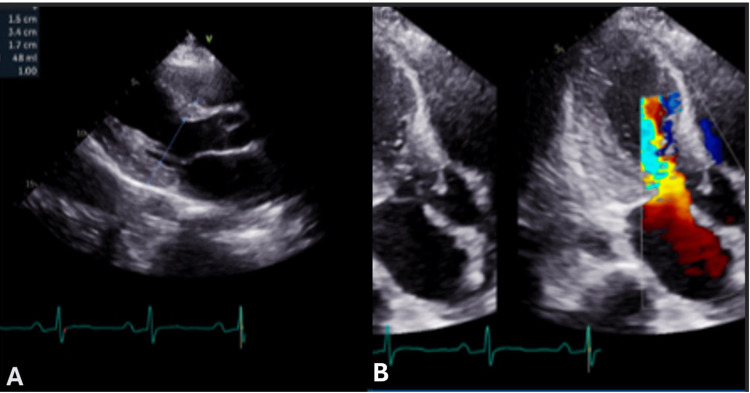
Parasternal long axis showing a septum and LV measurement of 1.5 cm consisting of LVH (A). Apical long axis showing an EF of 60%, diastolic dysfunction with increased mean LA pressure, and systolic anterior motion with LVOTO with a peak gradient of 111 mmHg (B). LA, left atrium; LV, left ventricle; LVOTO, left ventricular outflow tract obstruction
